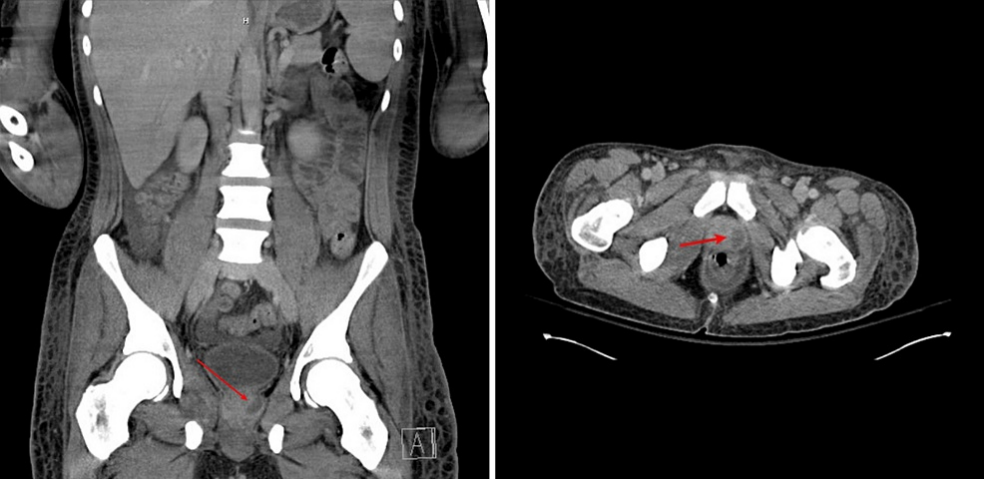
CT scan of the abdomen evidence (indicated by the arrow) left prostate abscess. CT: computed tomography.
